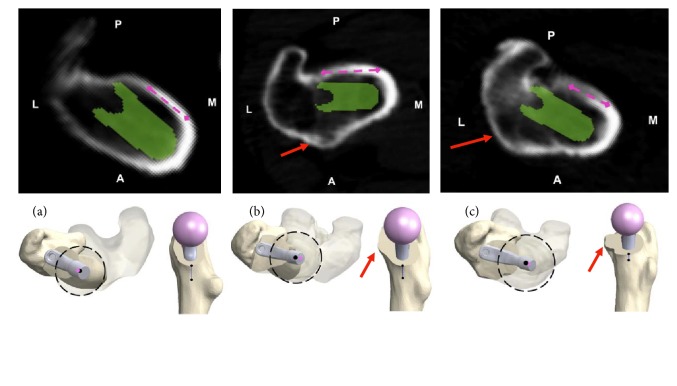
Case examples showing axial CT scan slices in the plane of the planned femoral neck osteotomy, with corresponding 3D reconstructions in the axial and sagittal planes (red arrows indicate anterior femoral neck osteophyte): (a) no anterior femoral neck osteophyte; (b) minor osteophyte; (c) extensive osteophyte.
